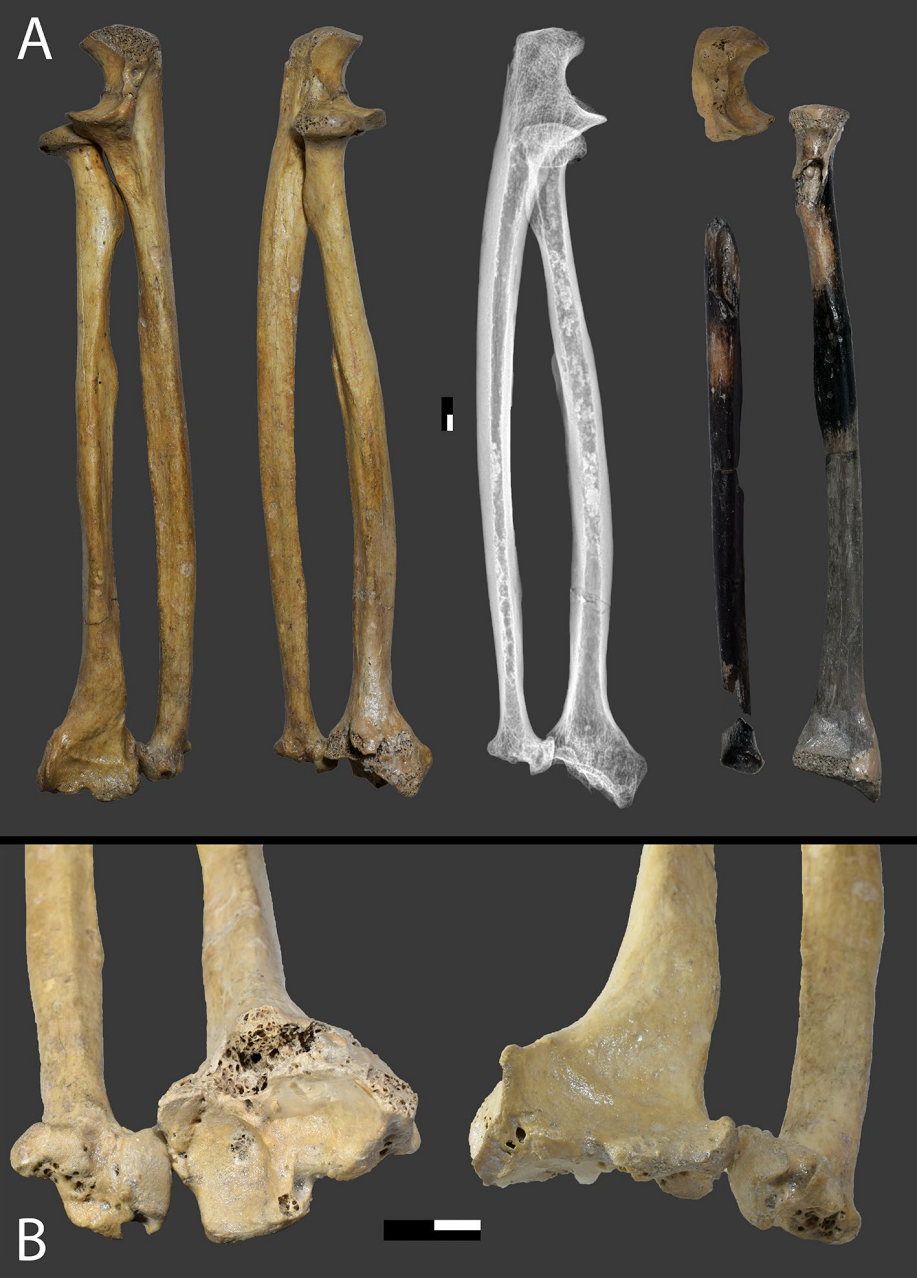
Morphological changes due to trauma in Nasino 2 forearm. (A) The right forearm of Nasino 2 in anterior radial, posterior radial view, and radiographic image of the articulated forearm, compared to the contralateral elements (frontal view). (B) Detail of the alterations in the radial and ulnar distal epiphyses. Scale: black bar indicates centimeters, white/black bar indicates 5 mm.
